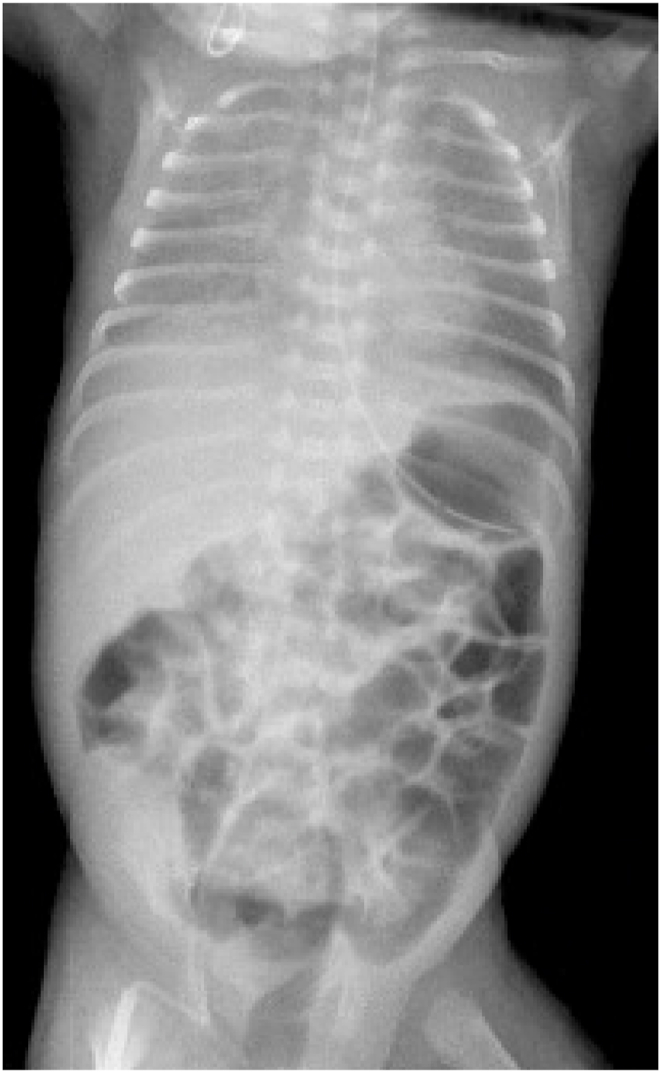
The initial X-ray shows ileus, which causes elevated diaphragms and atelectasis, (the ground glass appearance of the lungs).
